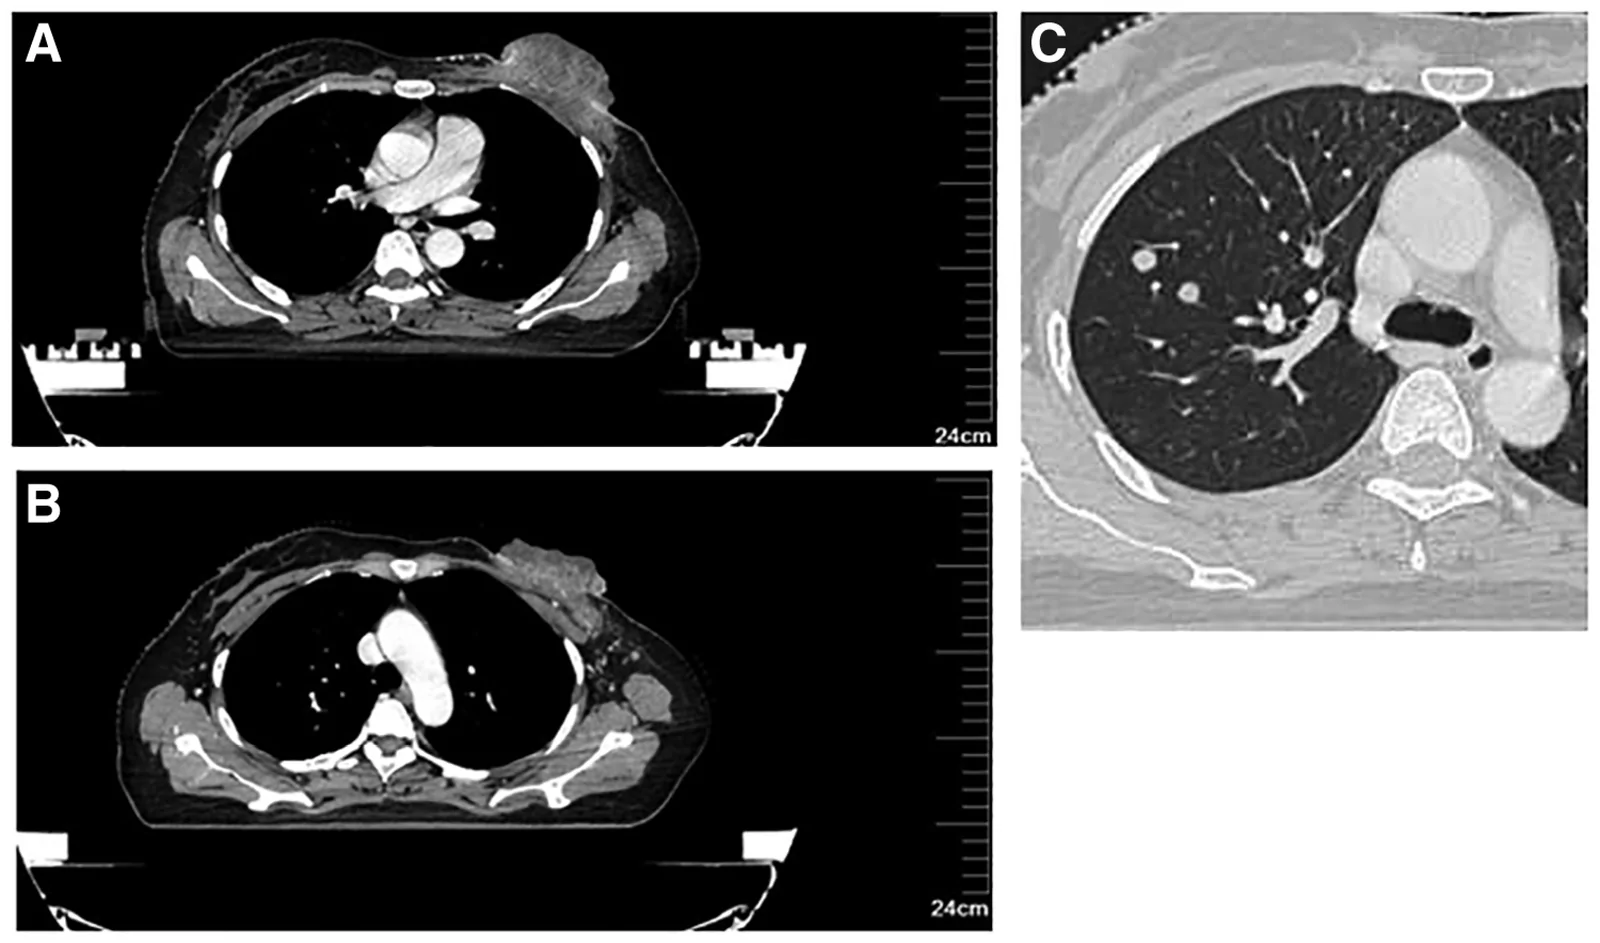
Contrast-enhanced CT revealed (A) a large tumor in the left breast; (B) a large neoplasm in the left breast at the aortic arch level; (C) metastatic lesions in the upper lobe of the right lung. CT = computed tomography.
